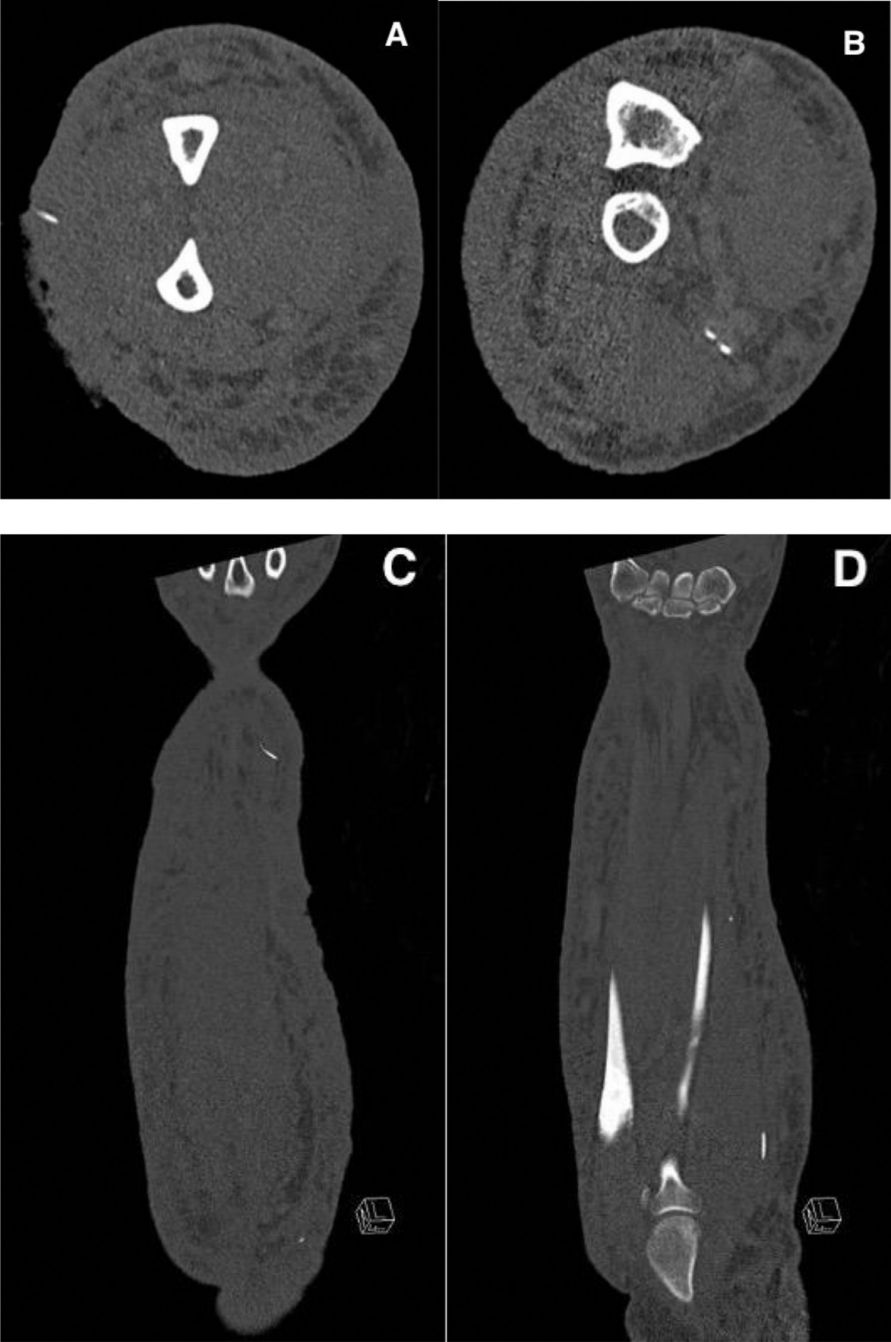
Contrast enhanced CT – Axial (A and B) and coronal (C and D) reformats demonstrating superficial and deep-seated uniform sized linear high-density objects, in keep with retained foreign bodies / needles related to IVDU along with area of skin ulceration. No abcess or osseous destruction was seen. Diffuse anasarca noted.
